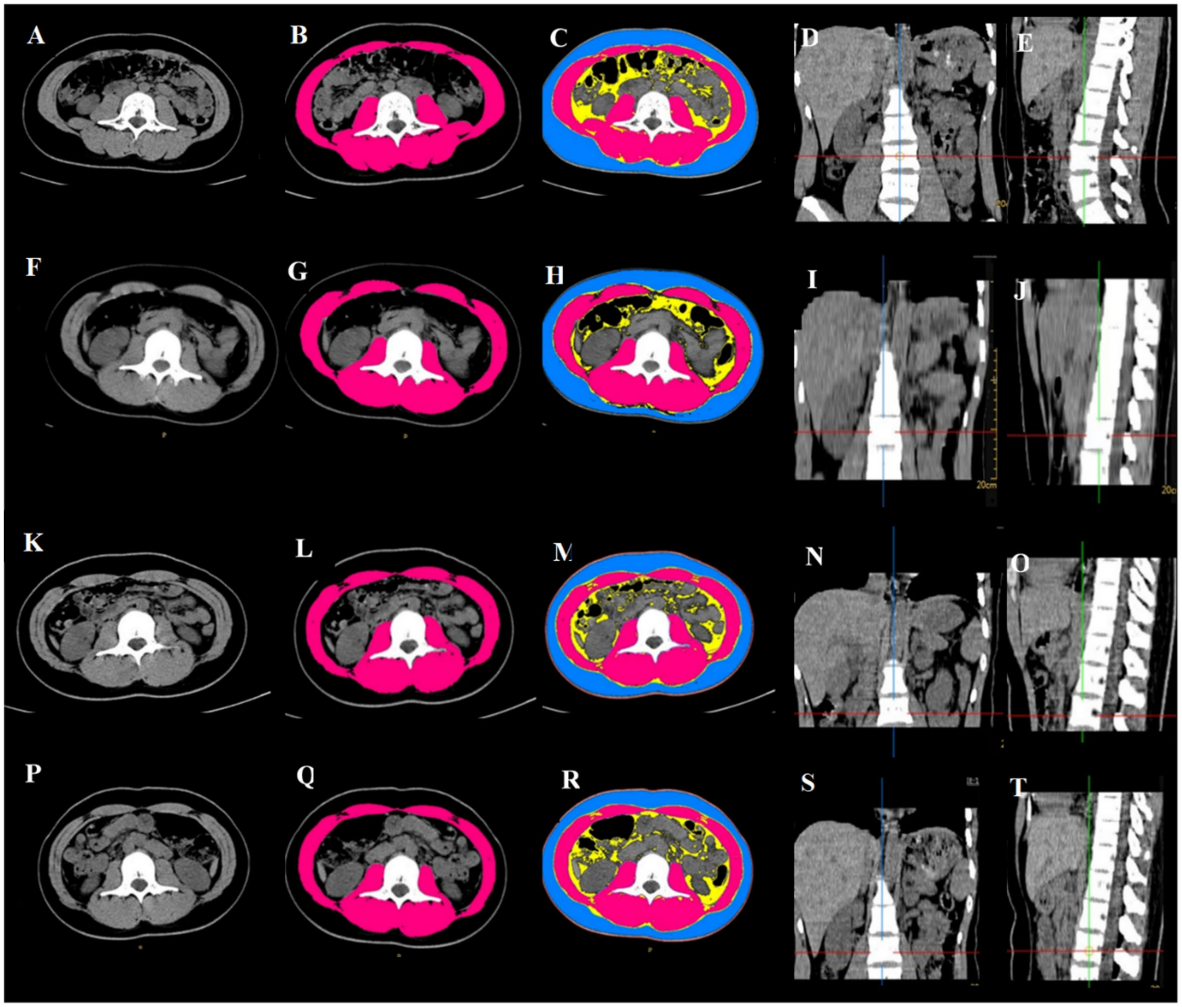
Abdominal CT scan showing changes in skeletal muscle mass at L3 after abdominal trauma in an 18-year female. (A–E) Within 14 days before surgery: SMA = 104 cm^2^, SMI = 38.81 cm^2^/m^2^; (F–J) post-trauma week 1: SMA = 83.5 cm^2^, SMI = 31.42 cm^2^/m^2^; (K–O) post-trauma week 2: SMA = 81.22 cm^2^, SMI = 31.42 cm^2^/m^2^; (P–T) post-trauma week 3: SMA = 78.03 cm^2^, SMI = 29.36 cm^2^/m^2^. (A,F,K,P) horizontal cross section at L3; (B,G,L,Q): skeletal muscle mass at L3 (red); (C,H,M,R) subcutaneous fat (blue), abdominal fat (yellow), skeletal muscle (red); (D,I,N,S) coronal plane, L3 is at the intersection of the red line and blue line; (E,J,O,T) saggital plane, L3 is at the intersection of the red line and green line.
